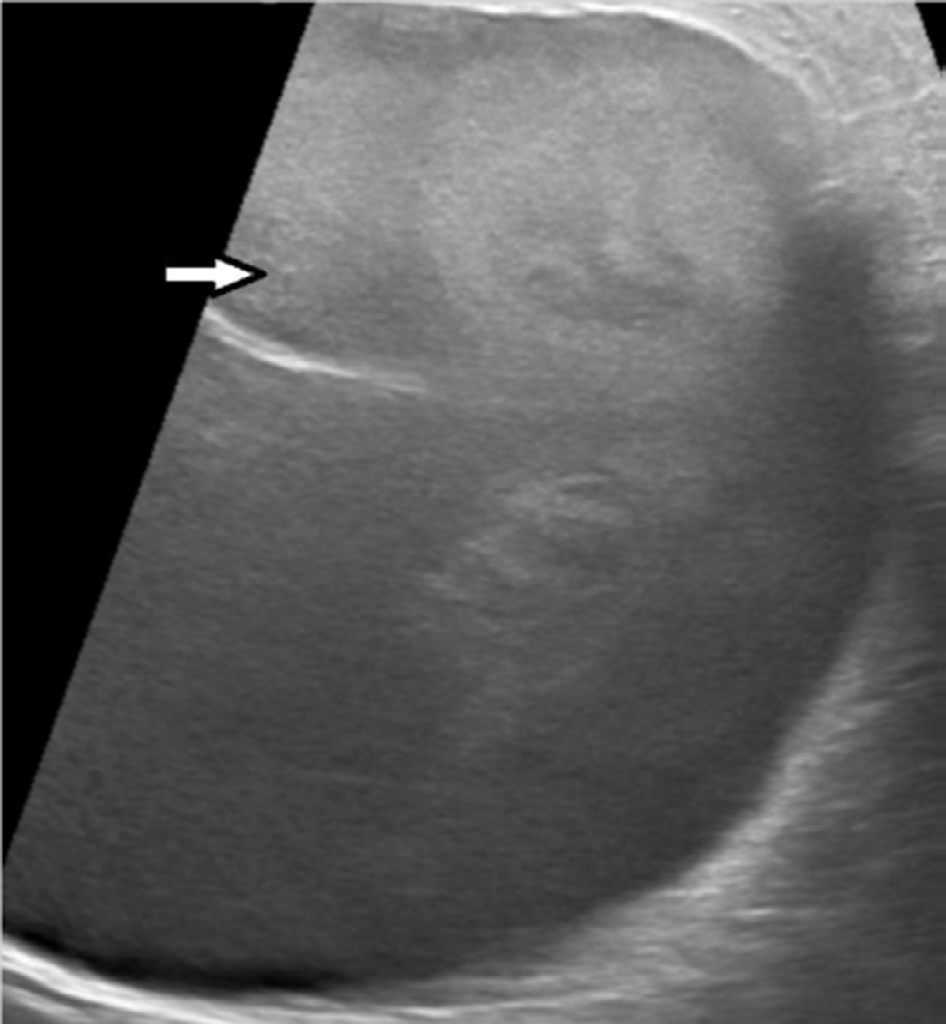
Abdominal ultrasonography showing a large well-defined, round cystic liver lesion with hypoechoic centre and an irregular wall
